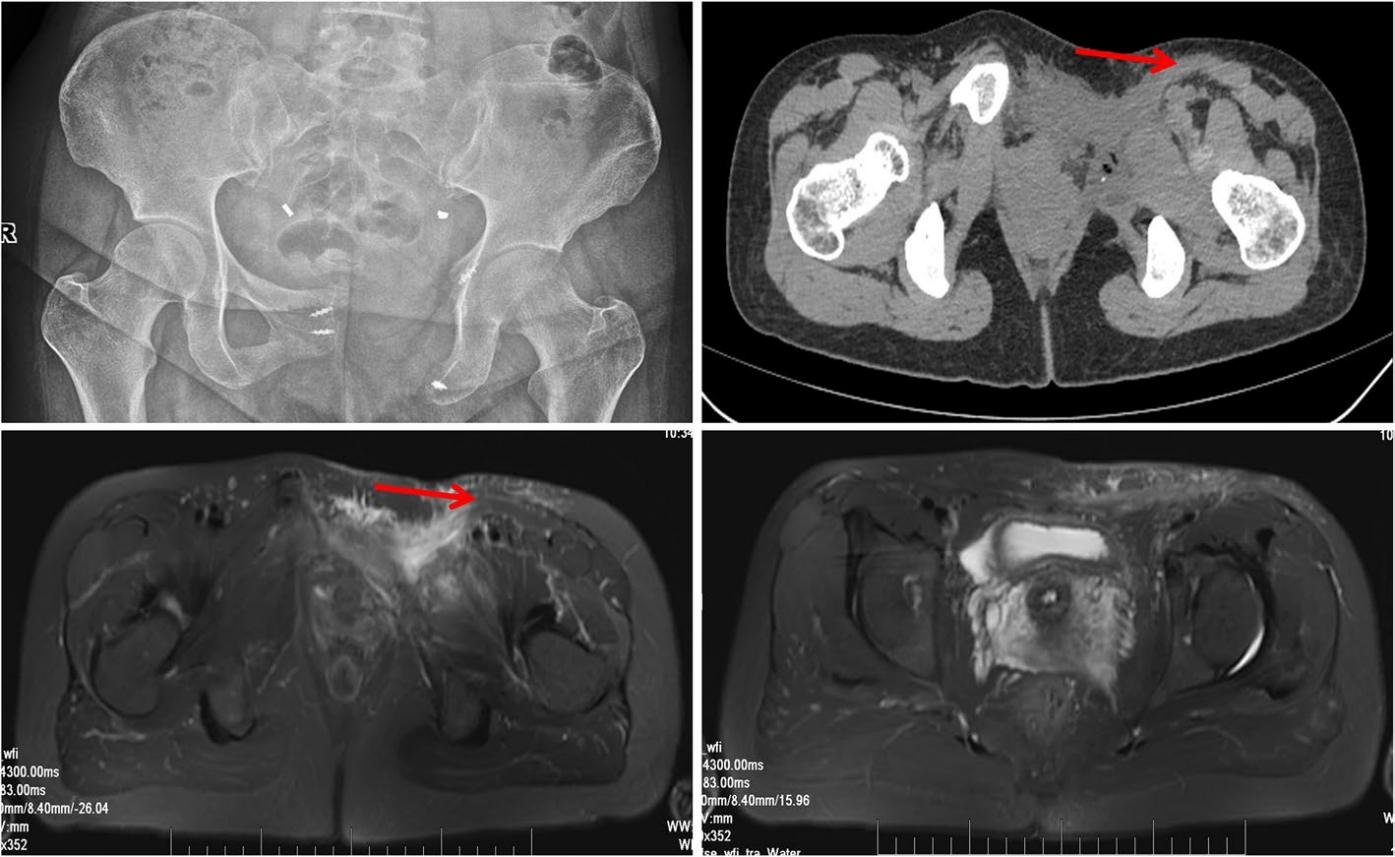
Pelvic bone imaging one year after surgery of case 3. X-ray (upper left) shows bony defect of pelvic ring after tumor resection. CT (upper right) and MRI scan (lower left) of the pelvic bone showing the pedicled sartorius flap as marked with red arrows. MRI scan of the pelvic bone (lower right) shows no sign of any herniation
